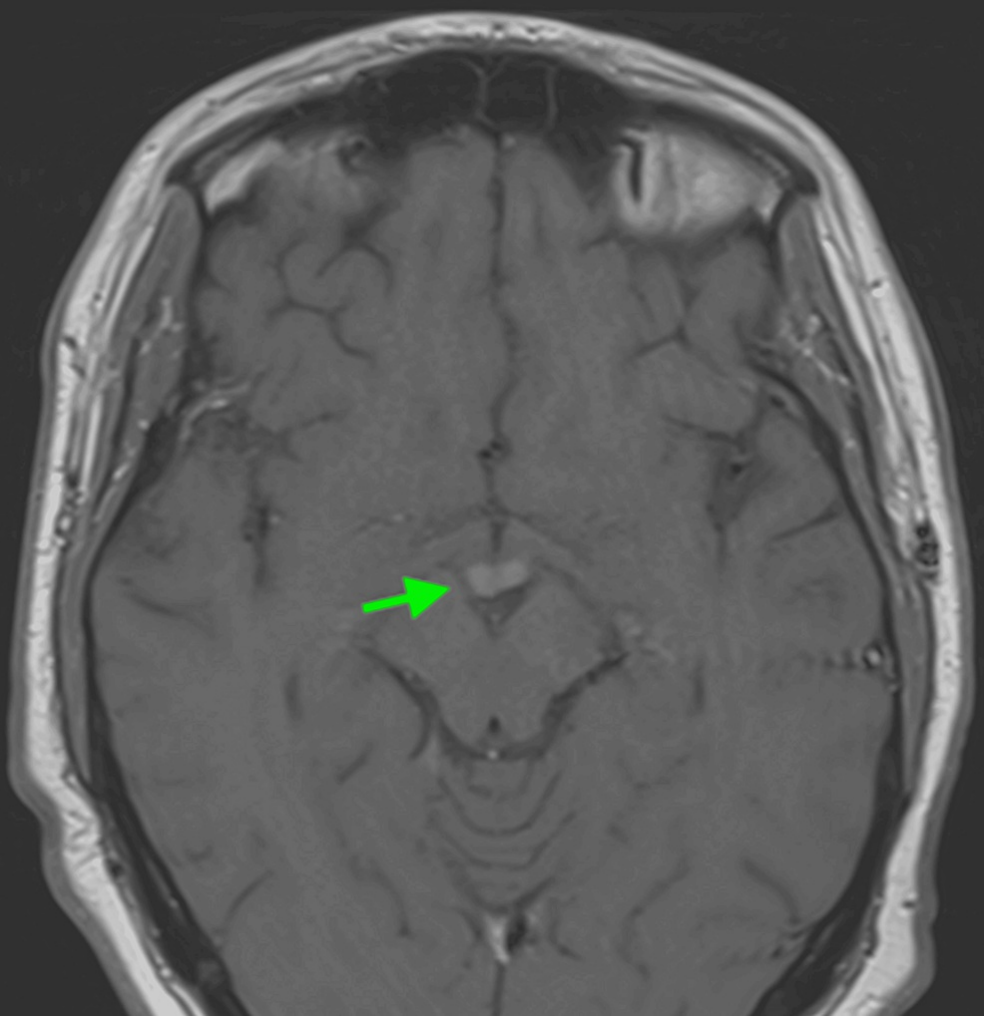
MRI brain with FLAIR imaging revealing edematous mammillary bodies (green arrow)
